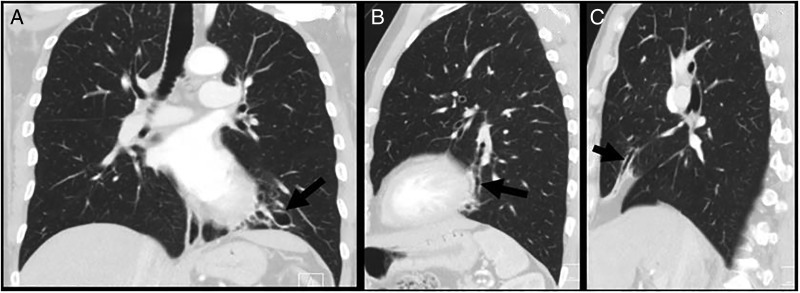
Coronal (A) and sagittal (B and C) CT reconstructions demonstrating right middle lobe (C) and left lower lobe (A and B) bronchiectasis (arrows) consistent with allergic bronchopulmonary aspergillosis.
